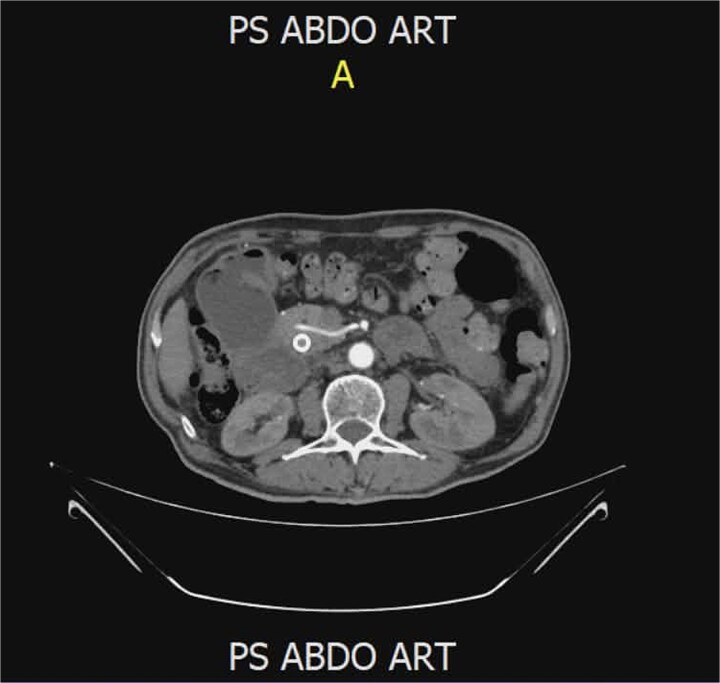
Preoperative CT scan showing the solitary right hepatic artery arising from the SMA and coursing through the pancreatic parenchyma.
